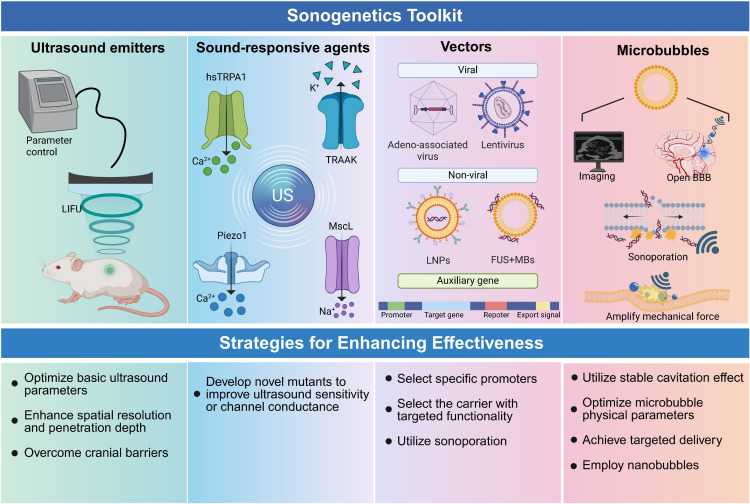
Sonogenetics toolkit and strategic pathways for enhancement. (1) Summarizes the essential components, including ultrasound emitters for parameter control, sound-responsive agents (e.g., hsTRPA1, Piezo1), viral and non-viral vectors for gene delivery, and microbubbles as amplifiers. (2) Outlines key optimization pathways to improve efficiency and safety regarding the aforementioned four aspects. Created with BioRender.com. BBB: blood-brain barrier; LIFU: low-intensity focused ultrasound; US: ultrasound.
